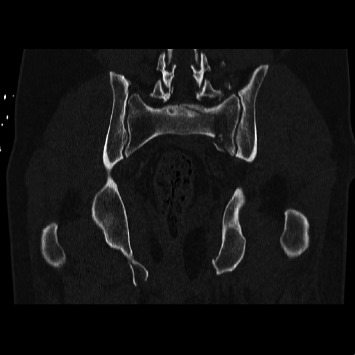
Coronal preoperative CT showing multiplanar sacral fracture resulting in spinopelvic discontinuity.
